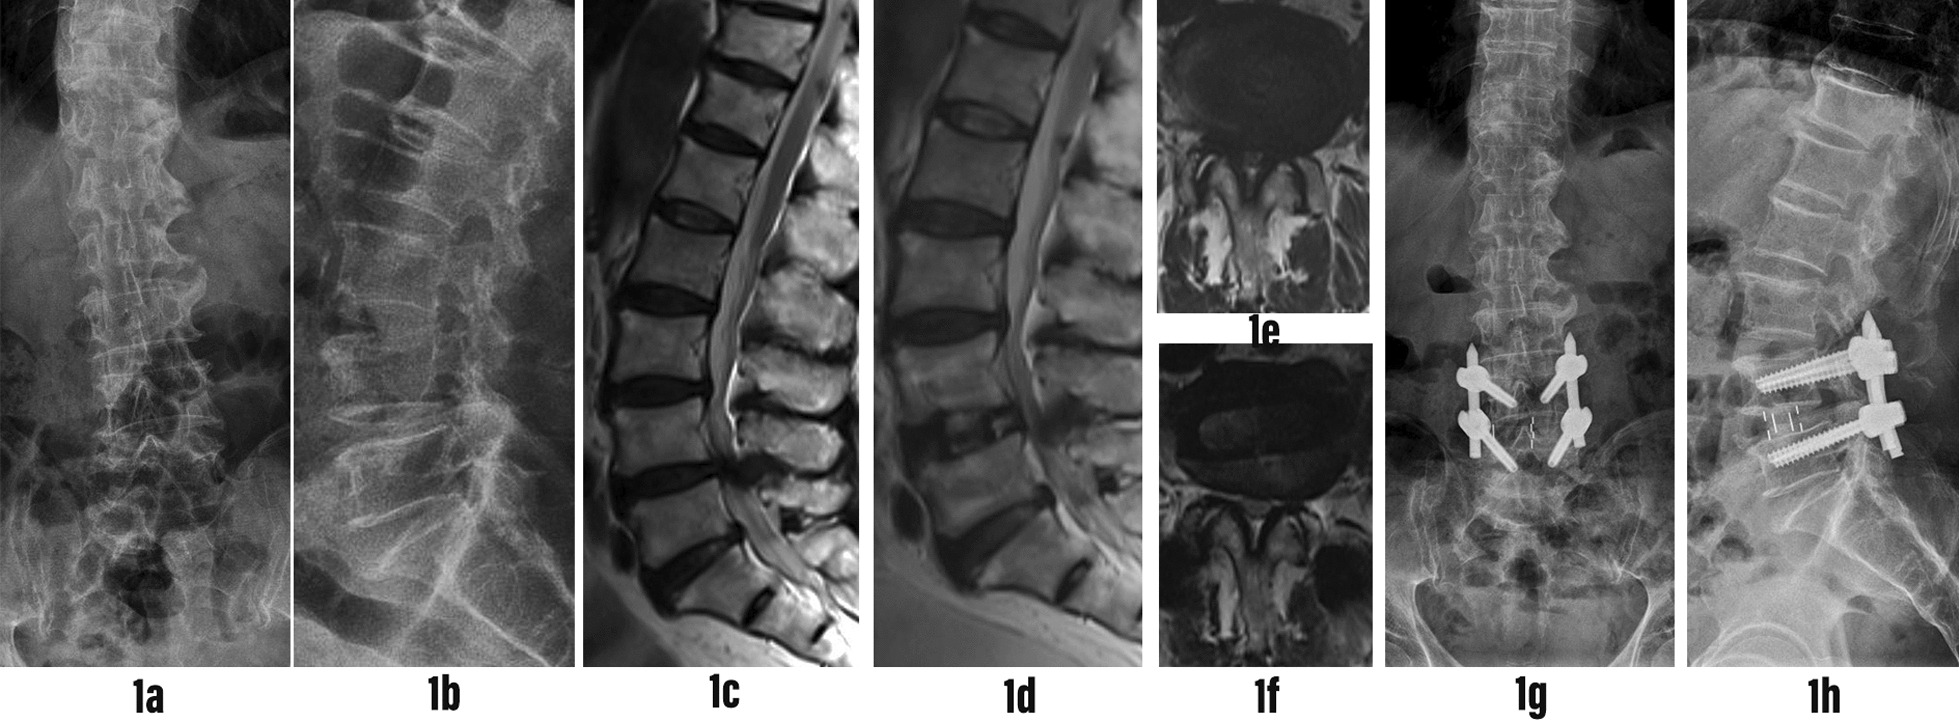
Typical case 1: A 70-year-old male patient presented with numbness of both lower limbs for more than 5 years, aggravated with left lower limb weakness for more than 1 month.Diagnosis of L4-5 spinal stenosis. L4-5 single segment OLIF combined with posterior L4-5 percutaneous internal fixation was performed. 1a, 1b Anteroposterior and lateral X-ray films of lumbar spine before operation showed lumbar degeneration. 1c Preoperative sagittal T2 MRI of the lumbar spine showed spinal stenosis in L4-5 segment, and RNRs above the stenosis plane. 1d Postoperative sagittal T2 MRI of the lumbar spine showed that RNRs still existed above the L4-5 surgical segment. 1e, 1f Axial T2 MRI of lumbar spine before and after operation showed that the dural sac CSA increased significantly after OLIF indirect decompression. 1g, 1h At the last follow-up, the X-ray film of the lumbar spine showed that the L4-5 pedicle screw was fixed and the fusion cage was placed in a good position
